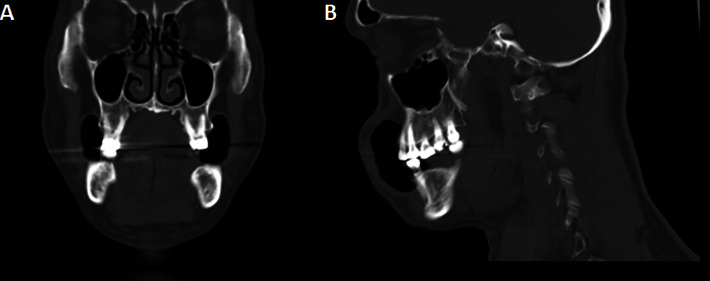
Coronal section of bone window medical computed tomography image shows no sign of bony invasion (A). Sagittal section of bone window medical computed tomography image shows no sign of bony invasion (B).
